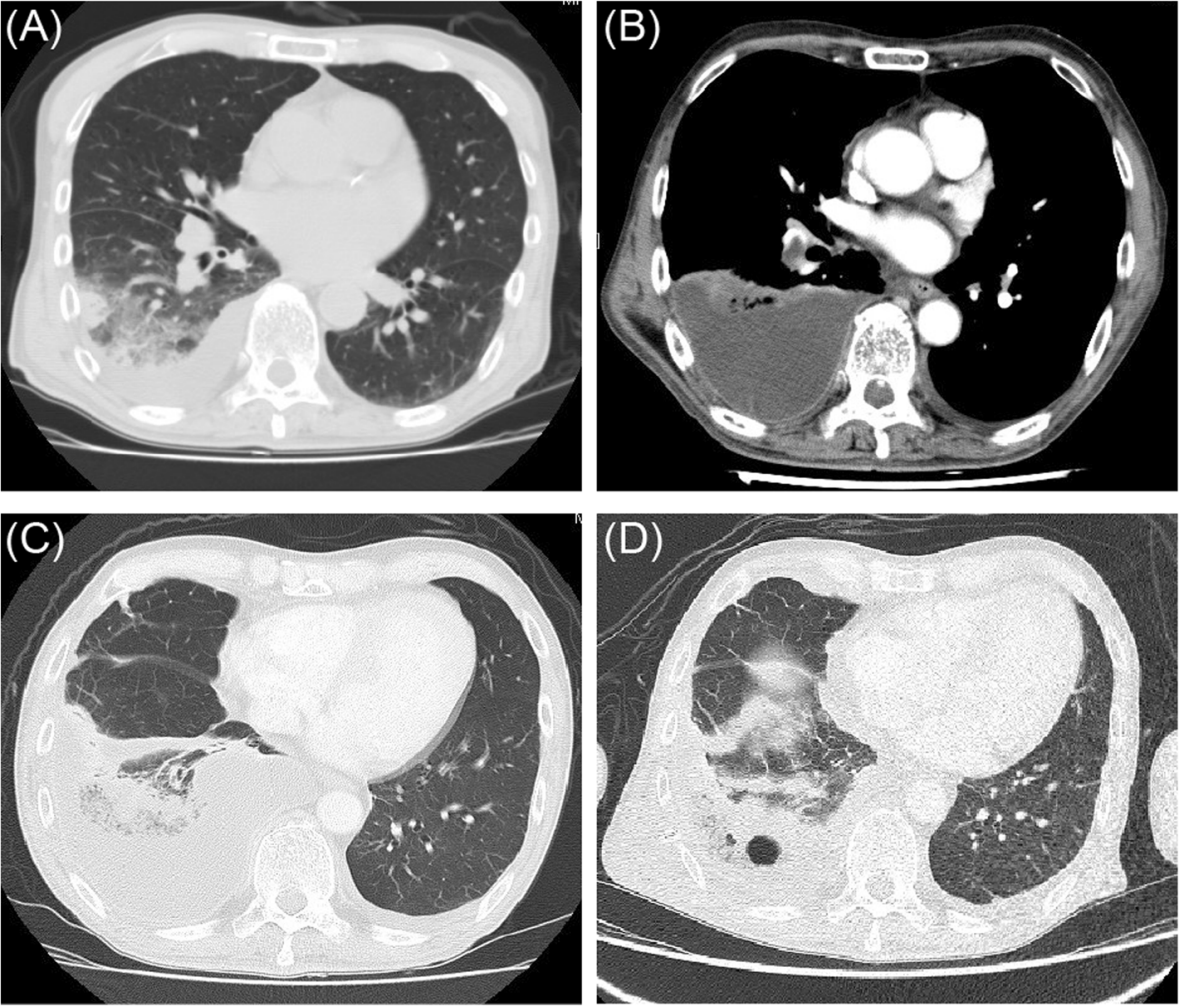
Axial sections on chest computed tomography (CT). a Plain CT at admission: infiltrative shadow in the right lower lobe and a small amount of right pleural effusion are visible. b Enhanced CT on post-admission day 7: an extensive lung embolism extending to the right basal pulmonary artery and A6 is seen. The pleural effusion had increased compared to that on initial CT. c Enhanced CT on post-admission day 7: persistent pulmonary infiltration is visible. d Enhanced CT on post-admission day 10: the pleural effusion decreased after thoracic drainage treatment. However, a cavitary lesion, 1.2 cm in maximum diameter, is visible in the infiltrative shadow in the right lower lobe
